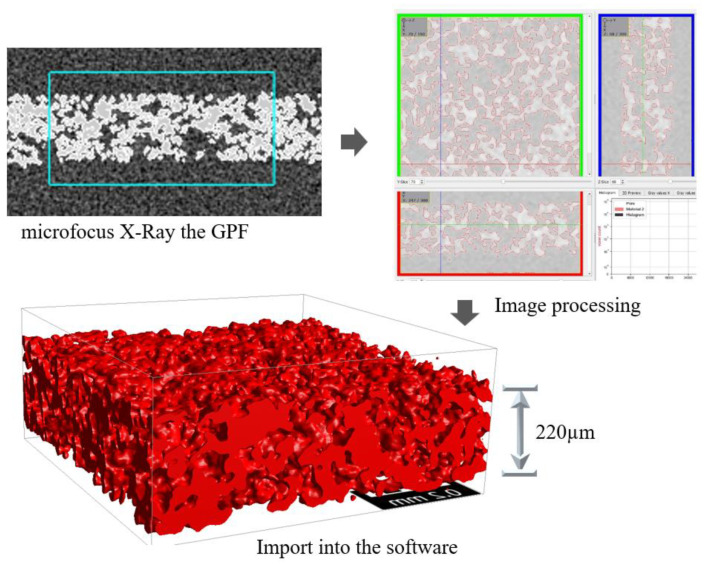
GPF model reconstruction process. GPF image obtained from microfocus X-ray (top left), GPF model after reducing noise by image processing (top right), and the final 3D GPF model (bottom).
